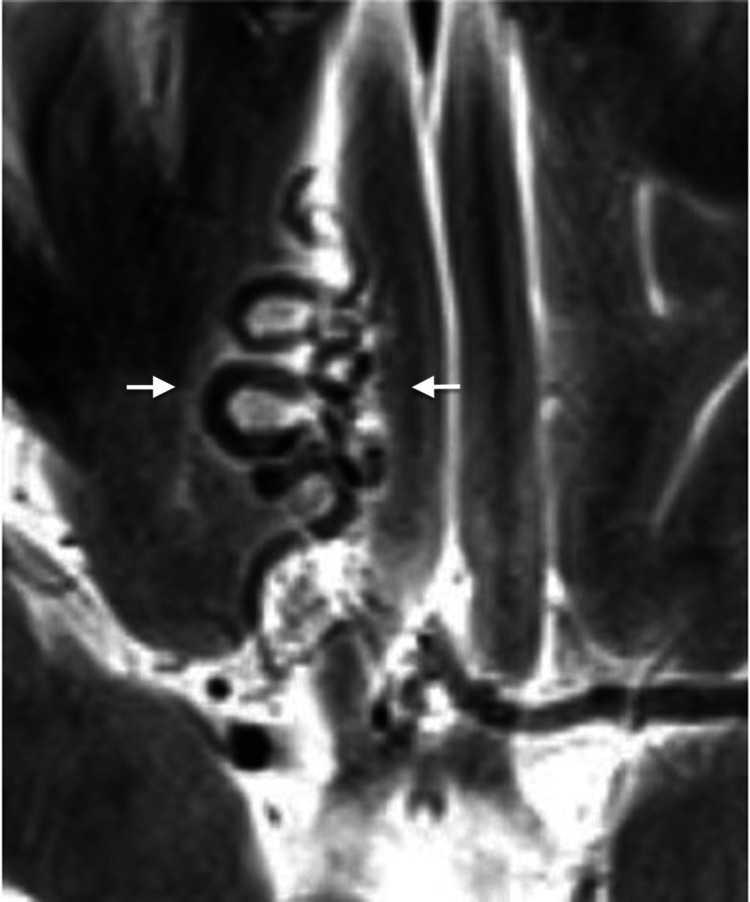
Axial T2-weighted MRI of the brain showing multiple tortuous flow voids in the right gyrus rectus extending toward the right basal ganglia (arrows), consistent with an arteriovenous malformation (AVM) or arteriovenous fistula.
